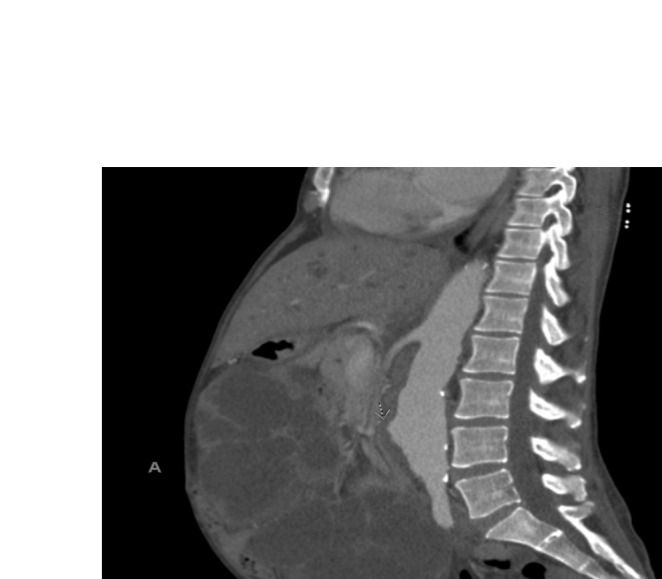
CT scan of the abdomen, sagittal view, showing IVC compression and mass effect.
